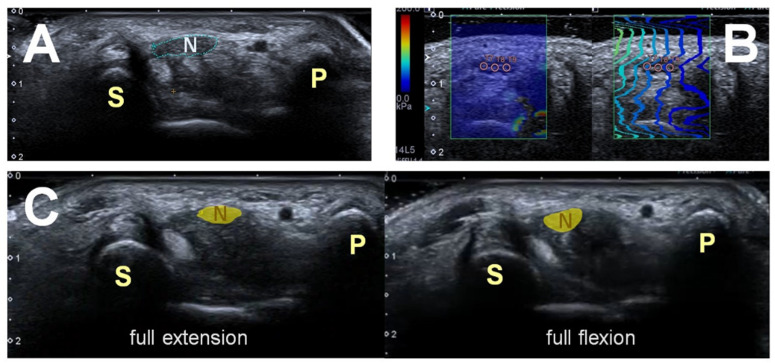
Measurement of ultrasound outcomes with (A) cross-section area (CSA), (B) shear-wave elastography (SWE), and (C) centroid position of the median nerve. (A) CSA of median nerve is measured at the carpal tunnel inlet level between scaphoid bone (S) and pisiform bone (P). (B) SWE of median nerve is measured at the carpal tunnel inlet level. Red color implies stiffer tissue and blue color implies soft tissue. Circular region is for quantitation of tissue stiffness in kilopascals (kPa) units. (C) Distance of the centroid position of the median nerve between the finger extension and full flexion position is recorded on ultrasound video. P = Pisiform bone. S = Scaphoid bone. N = Median nerve.
